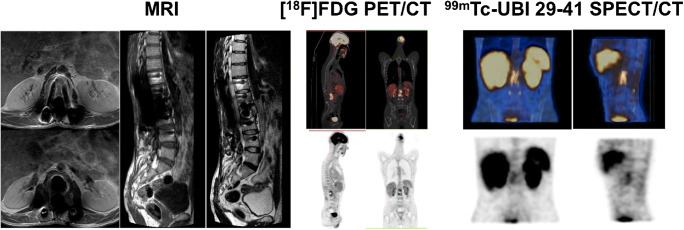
A 41-year-old man previously submitted to spinal fusion T12-L4 presented with lower back pain; ESR and serum CRP levels were markedly increased. Diagnosis of infection in this patient was based on biopsy. On MRI (left) the sagittal T1WI and T2WI of the thoracolumbar spine and axial T1WI with contrast of the lumbar spine demonstrate extensive posterior spinal implant extending from T12 to L4, which results in marked metal artifacts at the site of surgery. No MRI features to suggest spondylodiscitis can be identified. The [^18^F]FDG PET CT images (middle) show markedly increased tracer uptake, especially in the upper segments of the lumbar spine. The ^99m^Tc-UBI 29-41 SPECT/CT images (right) show markedly increased tracer uptake in the same segments exhibiting increased [^18^F]FDG uptake. Final diagnosis of postoperative spine infection was established in this patient on the basis of biopsy findings
